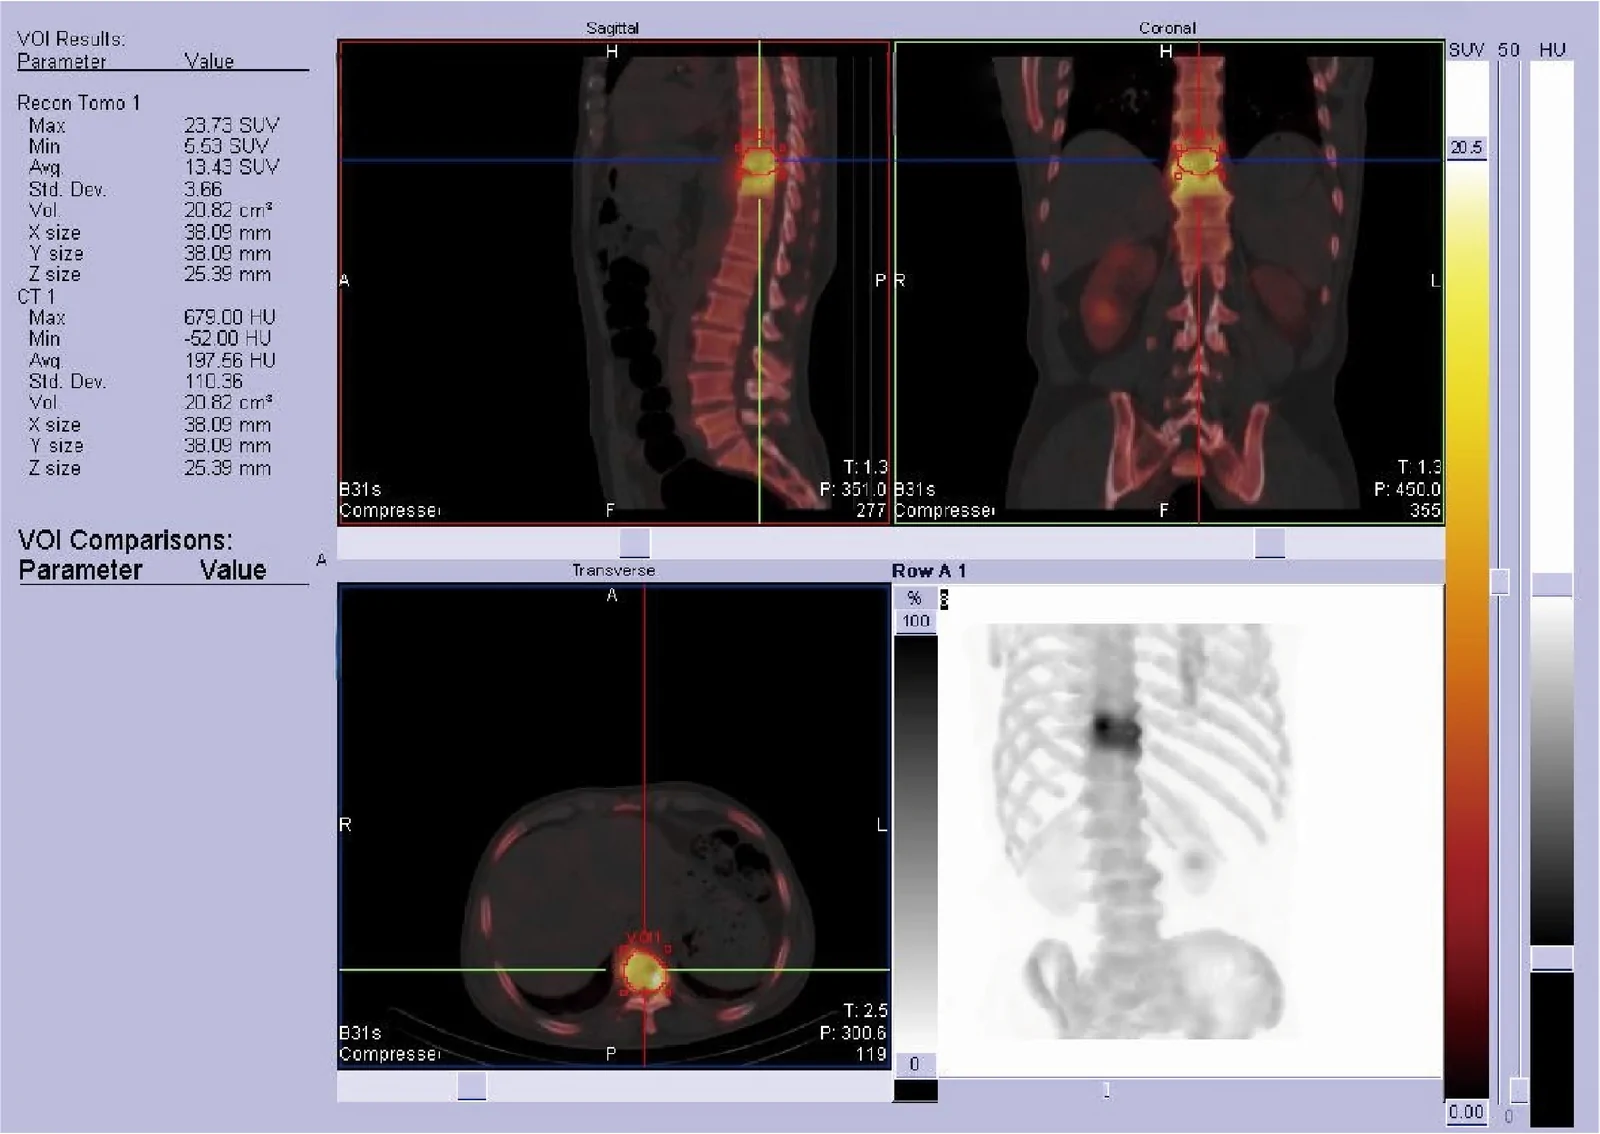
Quantitative SPECT/CT examination obtained in a patient with pyogenic spondylitis involving the T9 and T10 vertebra. Fused SPECT/CT images in the sagittal, coronal, and axial planes, together with the corresponding planar bone scintigraphy image, demonstrate increased radiotracer uptake in the involved T9 and T10 vertebral lesion.
